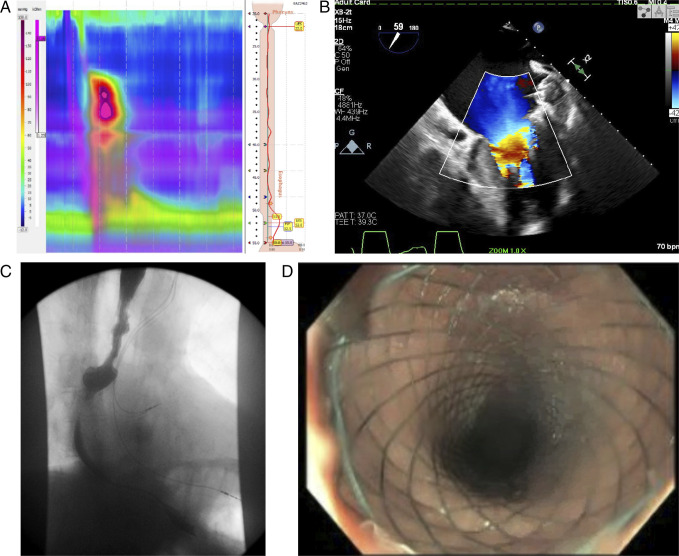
(A) Esophageal manometry showing findings concerning for grade III achalasia. (B) Echocardiogram revealing severe left atrial dilation and bowing of the interatrial septum. (C) Upper gastrointestinal series revealing esophageal dysmotility and pulsion diverticulum in the mid-esophagus just above the left atrium. (D) Post-esophageal stent placement.
